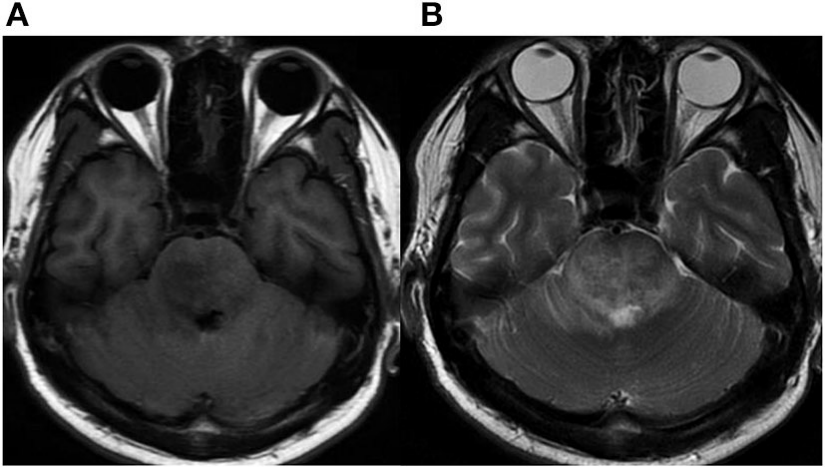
Initial MRI. (A) Axial MRI without contrast showing a hypointense signal on T1-weighted images in the right brainstem. (B) Intermediate signal on T2-weighted image surrounded by hyperintense signal of brain edema.
